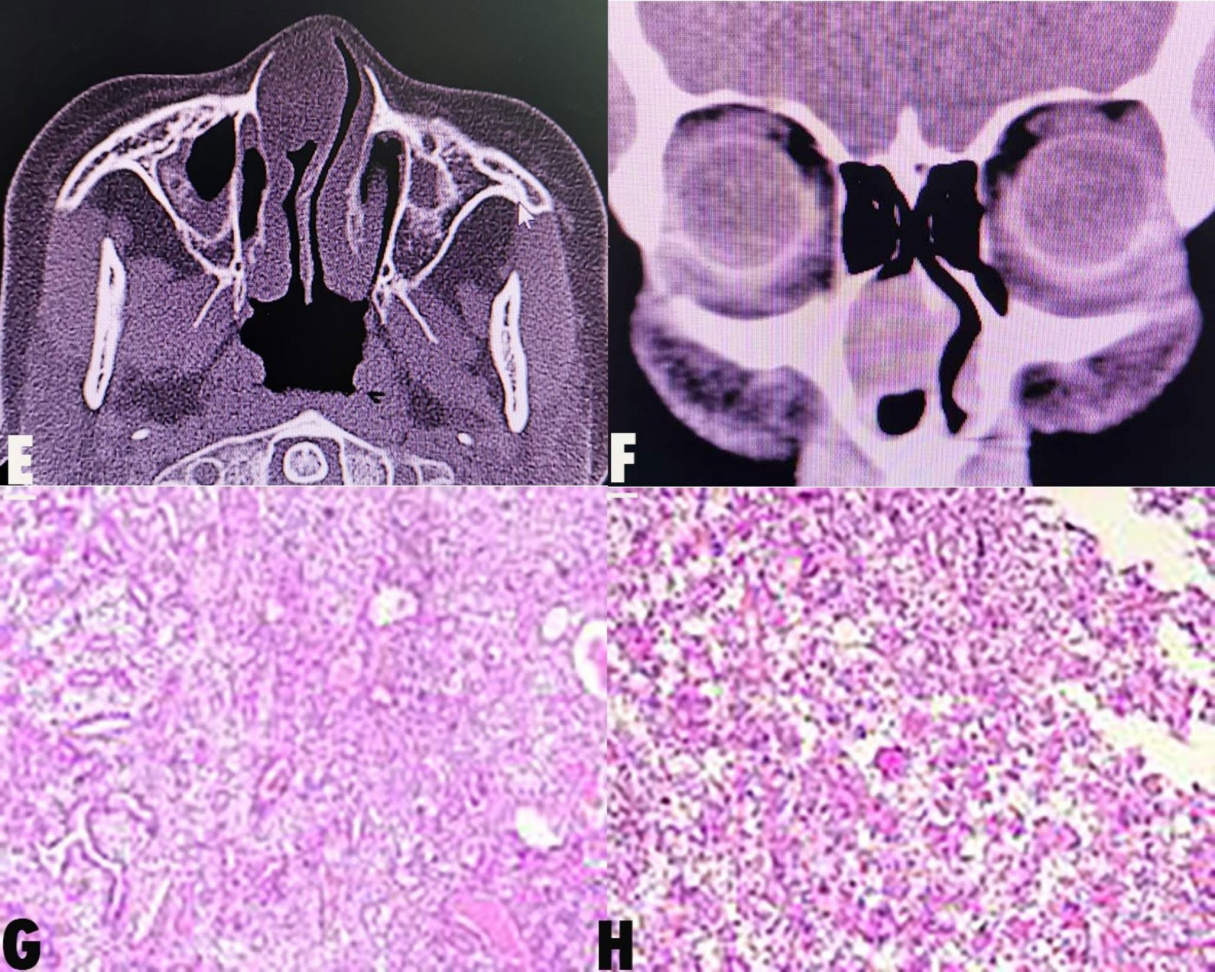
E and F suggested that a CT scan revealed an oval mass with soft-density in the right nasal cavity; G and H are the graphs of preoperative pathological biopsy report
